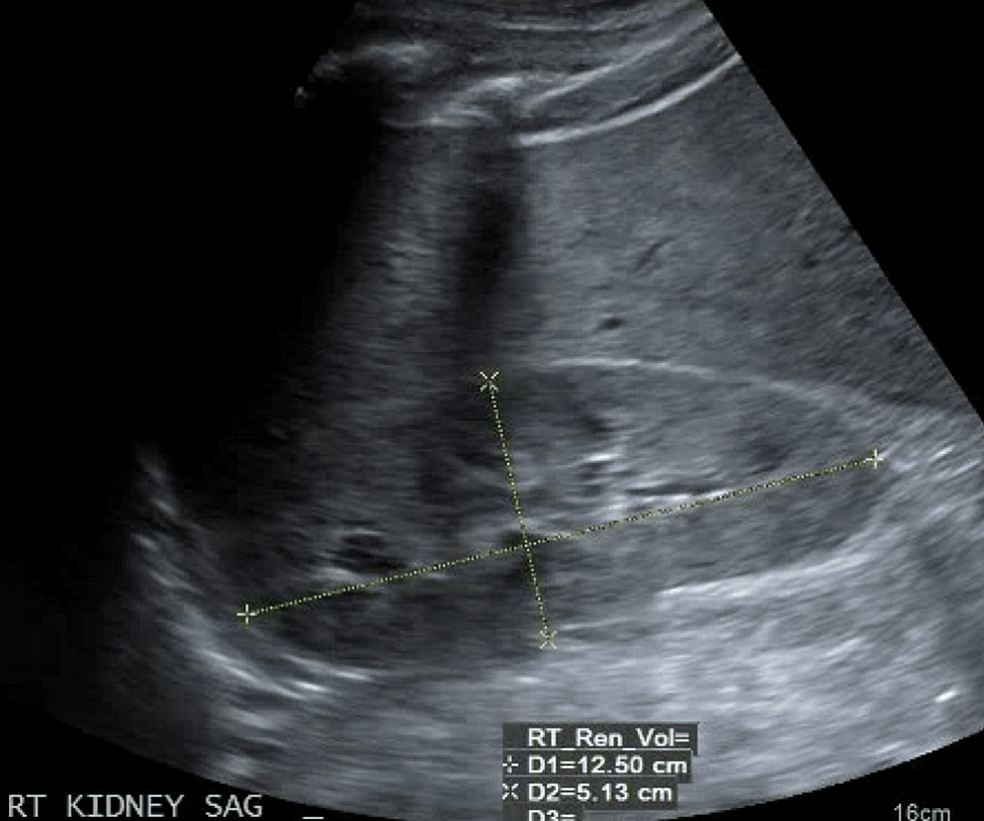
Renal ultrasound demonstrating increased echogenicity, suggestive of medical renal disease
